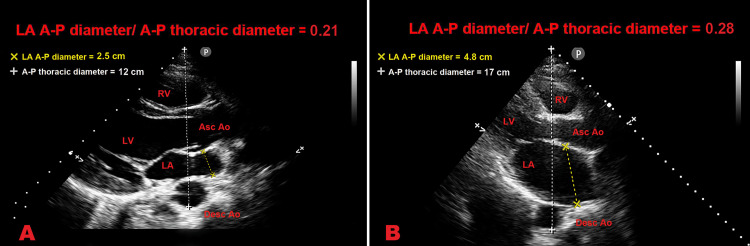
Examples of the LA A-P diameter/A-P thoracic diameter ratio obtained in individuals with concave-shaped chest wall (Panel A) and normal chest wall conformation (Panel B) respectively, included in the present study. In light of our findings, the magnitude of the LA A-P diameter/A-P thoracic diameter ratio obtained in the participant with a concave-shaped chest (≤0.25), as that measured in Panel A, identified an individual with a low probability of obstructive CAD and high probability of FP-ESE result. Ao, aorta; A-P, antero-posterior; Asc, ascending; CAD, coronary artery disease; Desc, descending; ESE, exercise stress echocardiography; FP, false positive; LA; left atrium; LV, left ventricle; RV, right ventricle.
